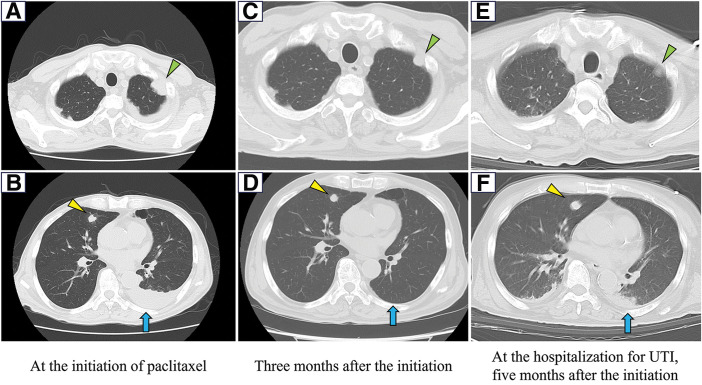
(A, B) A chest CT image taken on the 1st day of paclitaxel administration showed metastatic nodules in the left upper lobe (green arrowhead) and right upper lobe (yellow arrowhead), and pleural effusion (blue arrow). (C, D) A chest CT image taken at 3 months after the initiation of paclitaxel showed a shrinking nodule in the left upper lobe (green arrowhead) and a nodule with no change in the right upper lobe (yellow arrowhead), and decreased pleural effusion (blue arrow). (E, F) A chest CT image taken at the hospitalization for UTI, 5 months after the initiation of paclitaxel, showed the nodules with no change in the left upper lobe (green arrowhead) and right upper lobe (yellow arrowhead), and a small amount of pleural effusion (blue arrow). UTI, urinary tract infection
